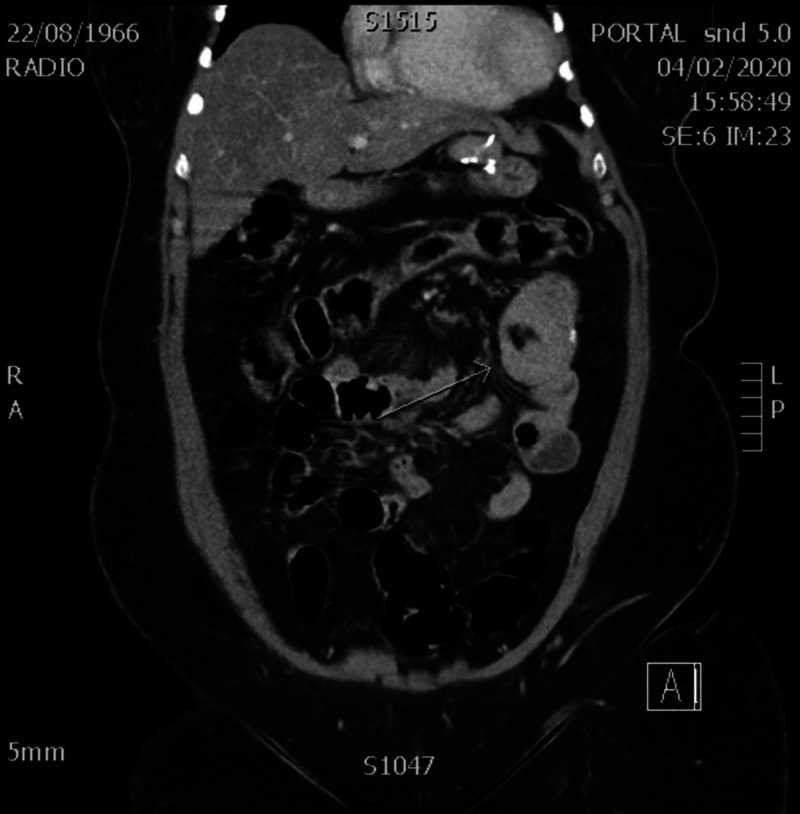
Coronal view of the CT scan showing intussusception at level of jejuno-jejunal anastomosis
